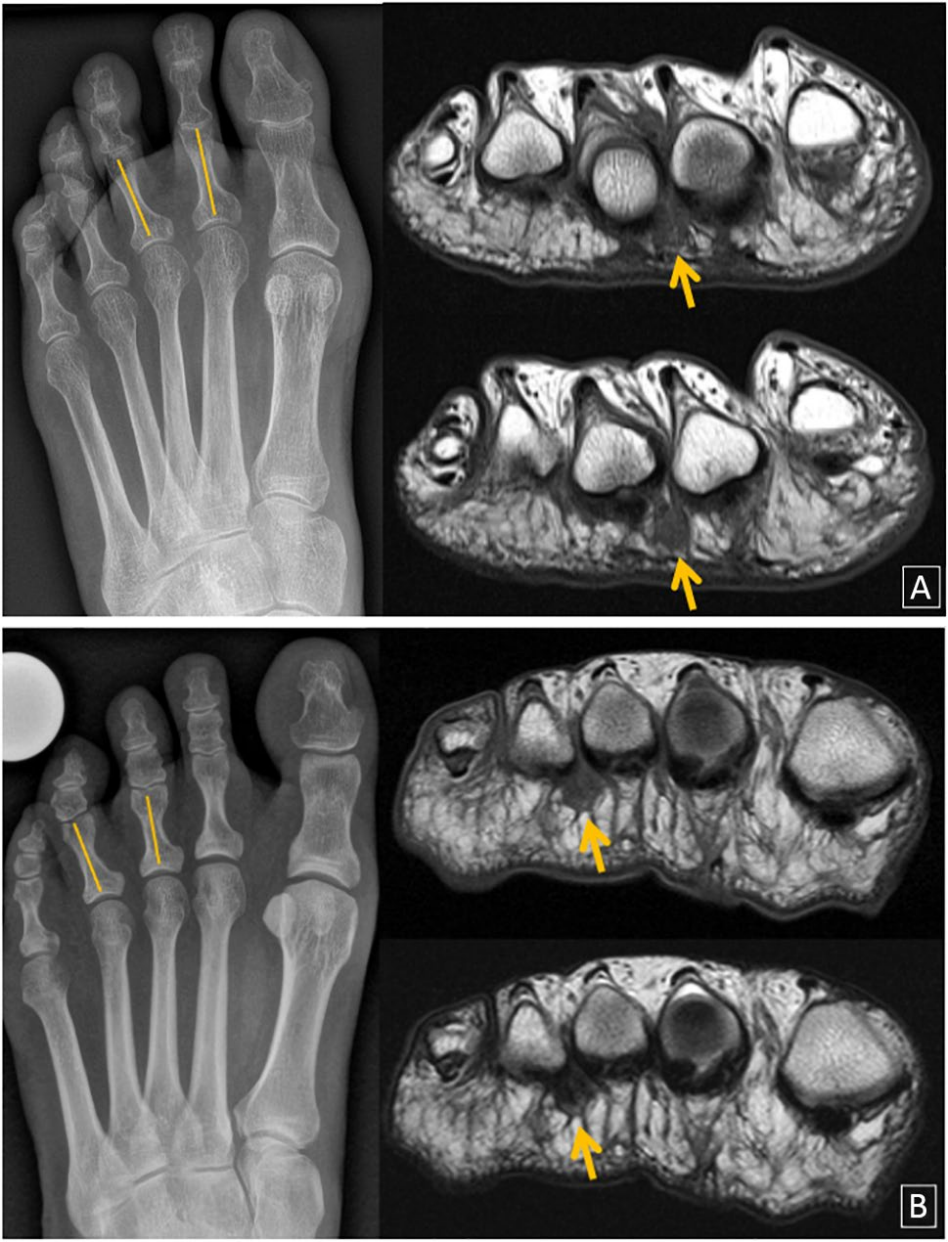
Positive V-sign. A Fifty-two-year-old woman with positive 2/3 V-sign on radiograph (left). Coronal T1-weighted (up) and T2-weighted (down) spine-echo MR images demonstrate the presence of a typical T1/T2 hypointense Morton’s neuroma (orange arrow). B Forty-nine-year-old woman with positive 3/4 V-sign on conventional radiograph (left) and the corresponding MR images (right) showing Morton’s neuroma (orange arrow)
